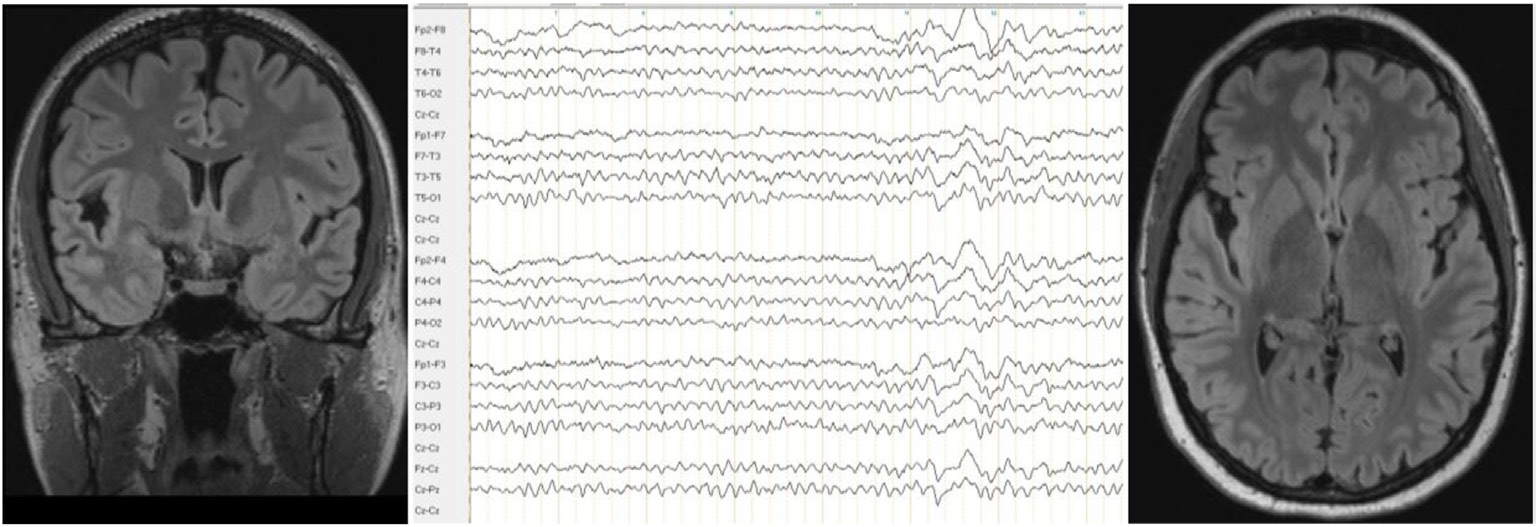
The cerebral magnetic resonance imaging showed mild temporolateral atrophy, and the electroencephalogram showed intermittent generalized slow waves.
